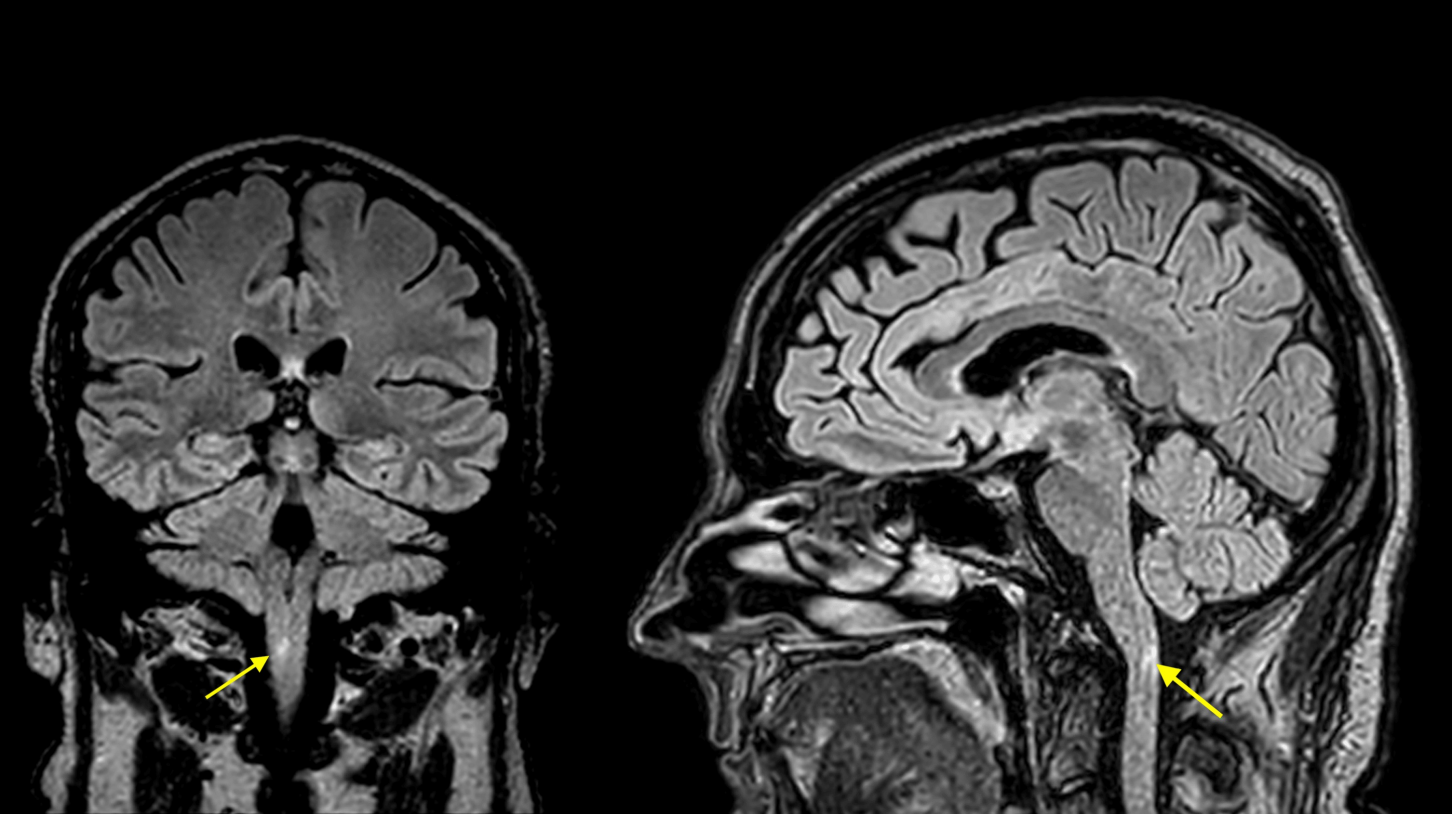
FLAIR MRI demonstrating demyelination in the area postrema Coronal and sagittal fluid attenuated inversion recovery (FLAIR) MRI views showing a hyperintense lesion in the caudal medulla (yellow arrows), consistent with demyelination of the area postrema. This circumventricular organ acts as a chemoreceptor trigger zone for emesis by detecting circulating toxins. Its involvement likely explains the patient’s persistent vomiting.
